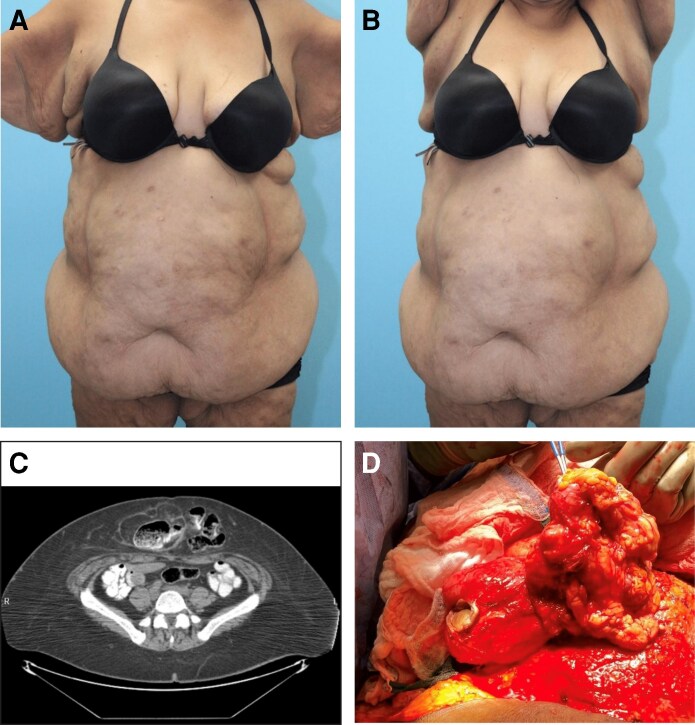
Forty-five-year-old woman with abdominal lipodystrophy and umbilical hernia, 6 months postop. (A) Preoperative photo, anatomic position. (B) Preoperative photo, arms out position. (C) Axial computed tomography showing umbilical hernia. (D) Intraoperative photo.
